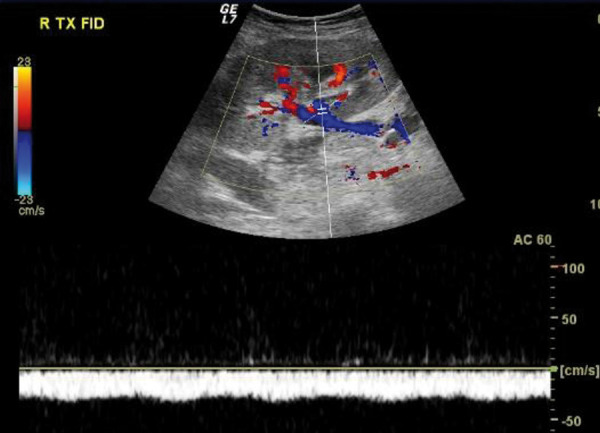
Doppler ultrasonography on day 5 after balloon catheter and aspiration venous thrombectomy showing Doppler signal on the renal vein with continued diastolic flow.
